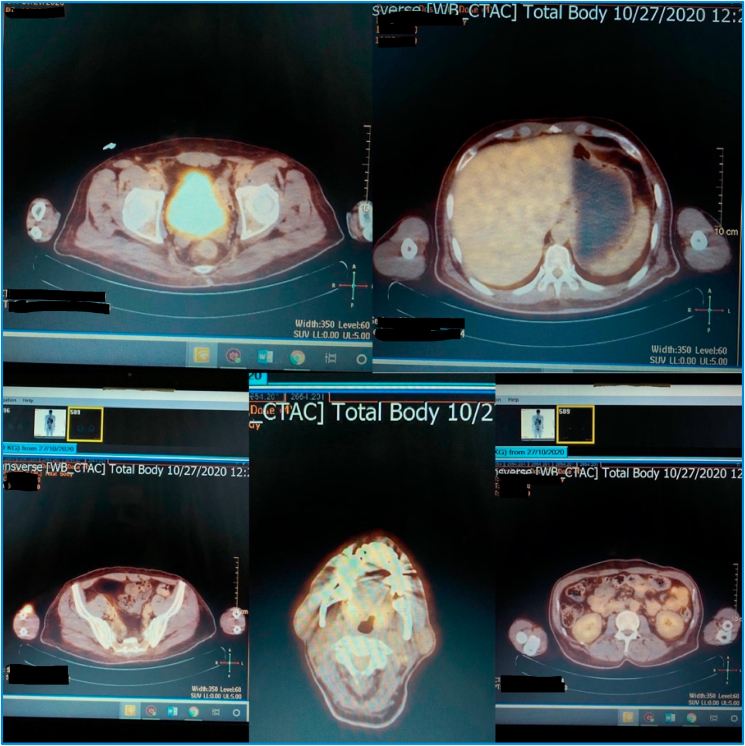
PET CT-scan showing hypermetabolic sub-centimetric cervical lymph nodes in the right side, the mediastinum, para-spinal region and abdominal and pelvic regions.
